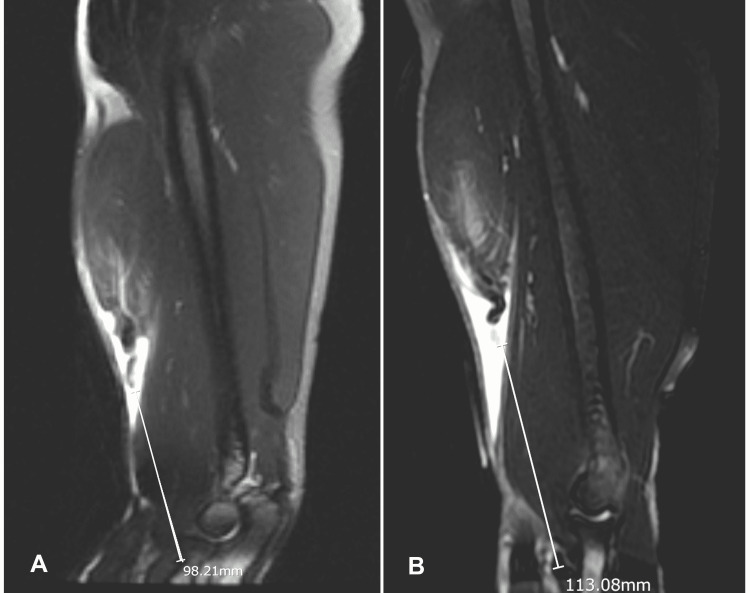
Sagittal T2 MRI images of right (A) and left (B) humerus Chronic full-thickness rupture of the distal biceps tendon with a gap of about 10 cm on the right (A) and 11 cm on the left (B).
